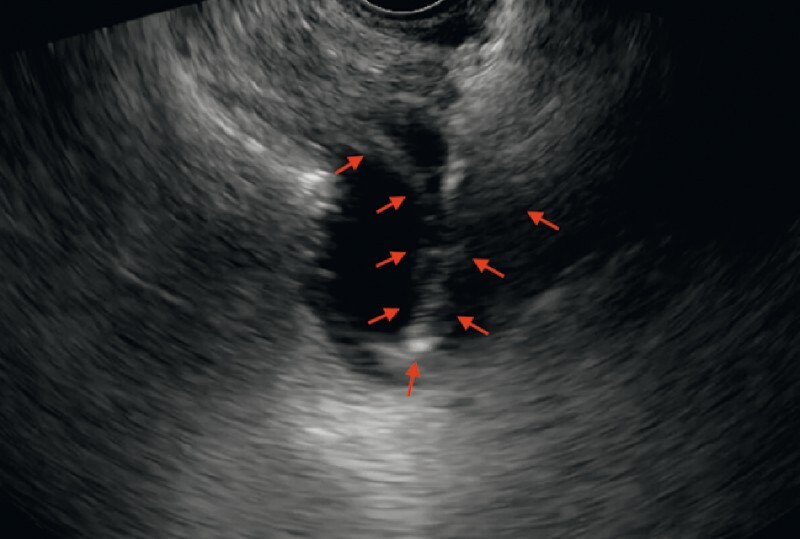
Endoscopic ultrasound (EUS) image showing the tent-like sign of the bowel wall (arrow) that resulted from the tip of the delivery system of the lumen-apposing metal stent incompletely penetrating the targeted jejunum during EUS-guided gastroenterostomy.
